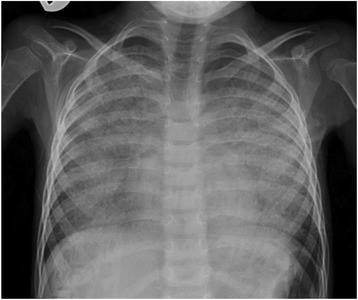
Chest X-ray showed widespread bilateral air-space disease
